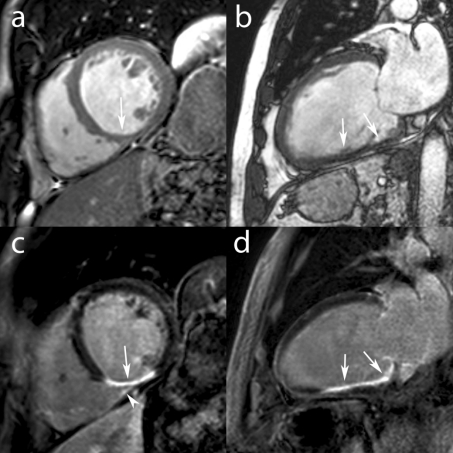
MRI study in a 58 year old male recently diagnosed with dilated cardiomyopathy (LV EF 20%). Still midventricular short-axis (a) and vertical long-axis (b) images of cine MRI show a remodeled, dilated LV and inferior wall thinning (*arrows*, a,b). Late ce-MRI in the same imaging planes show transmural enhancement of the basal and mid inferior wall (c,d) and of the mid inferomedial RV suggesting an old inferior MI with RV involvement (*arrow head*). Coronary angiography showed trivessel disease with RCA occlusion.
